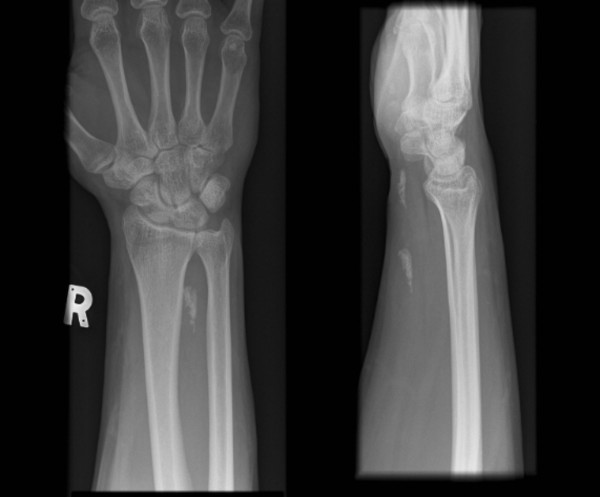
Radiographs of Right wrist demonstrating Calcific tendonitis of Flexor Carpi Ulnaris.
